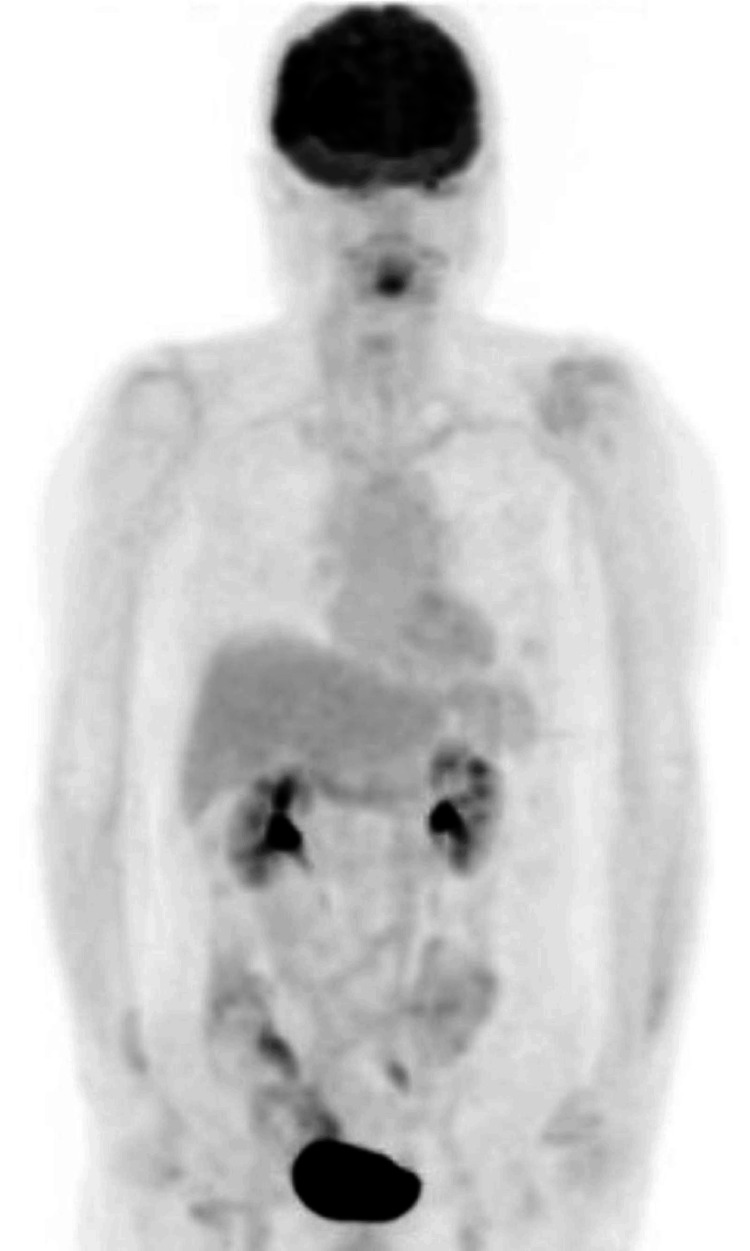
PET-CT image at relapse showing no abnormal accumulation of fluorodeoxyglucose in the thoracic and abdominal regions PET, positron emission tomography; CT: computed tomography
